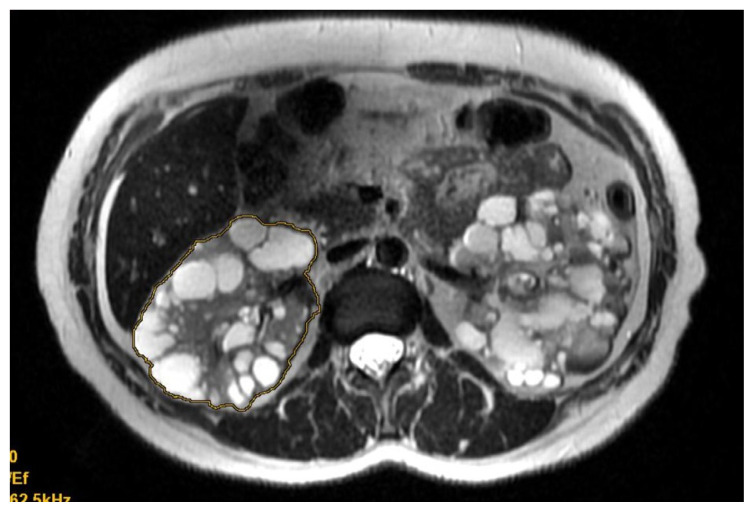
MBTM for TKV of ADPKD: kidney boundaries manually drawn on axial plane T2-weighted MRI.
